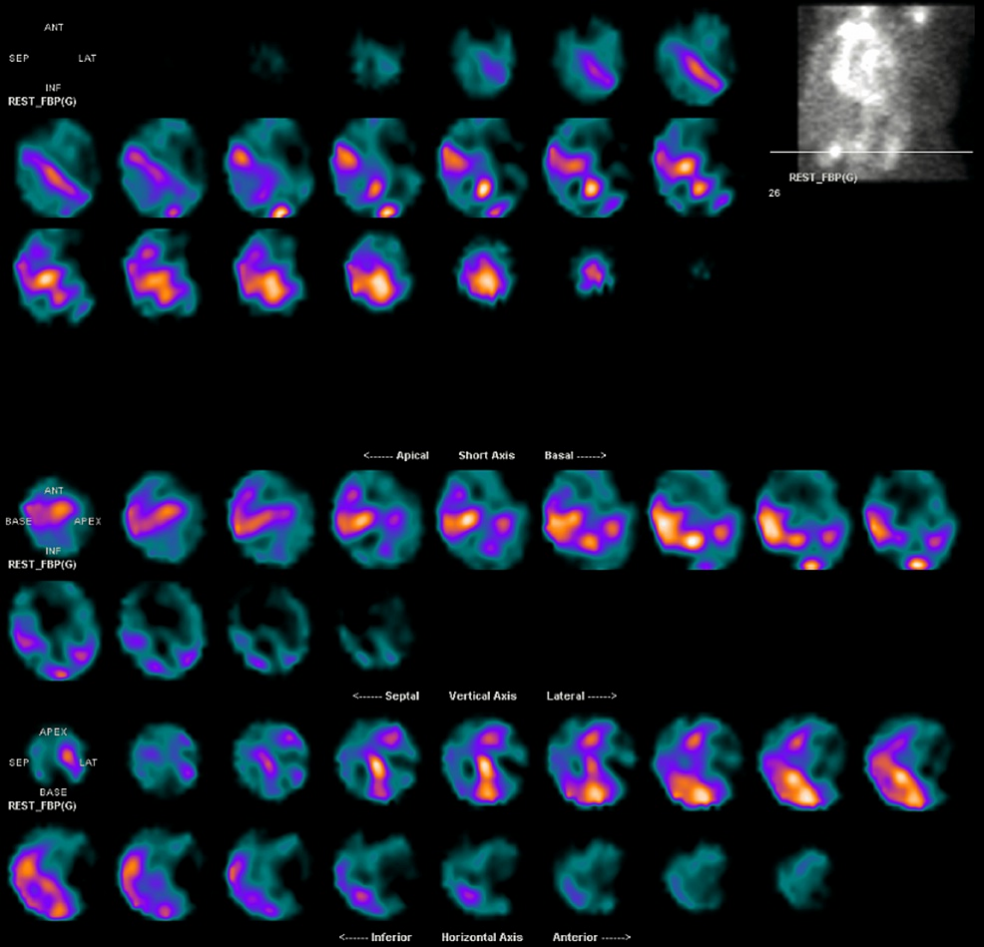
Single-photon emission computed tomography (SPECT) imaging of the myocardium Three-dimensional display confirming uptake of radioactive tracer in the myocardium.
